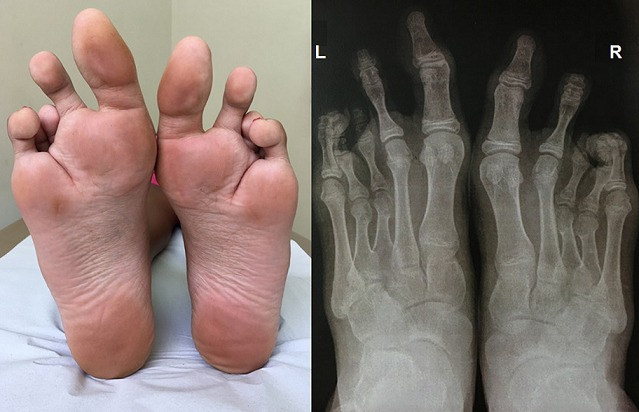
Plantar deep cleft and radiography of the feet showing third and fourth metatarsal shortening along without osteodystrophic features
